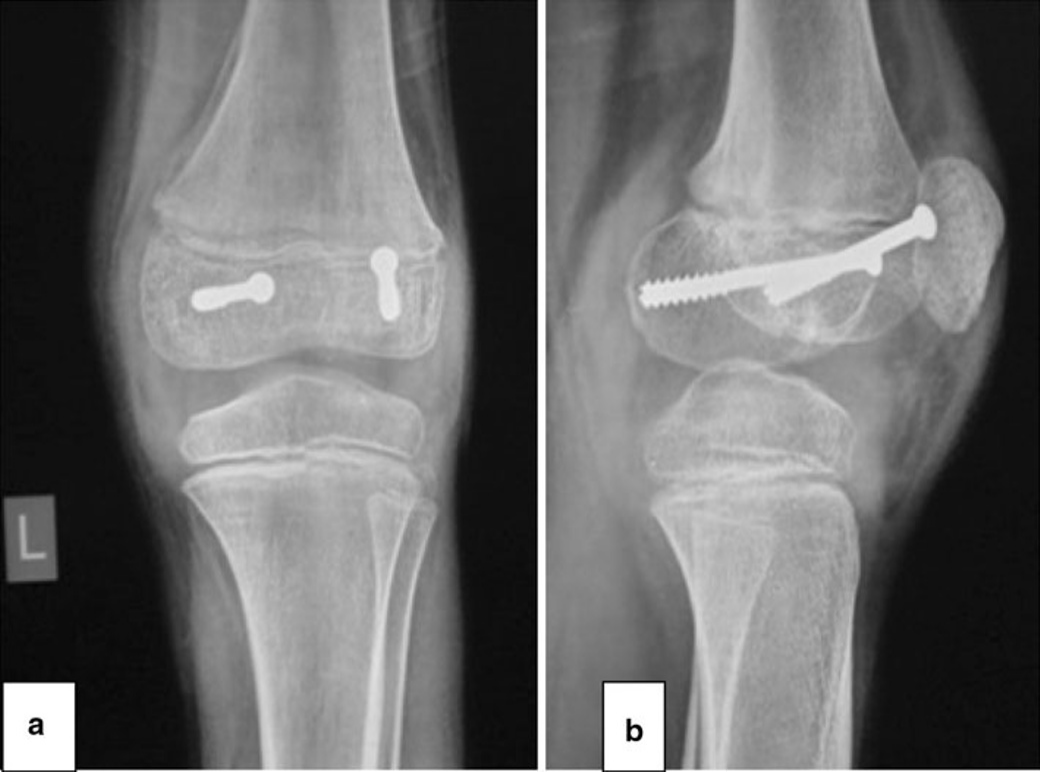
Anteroposterior a and lateral b radiographs showing the united fracture
